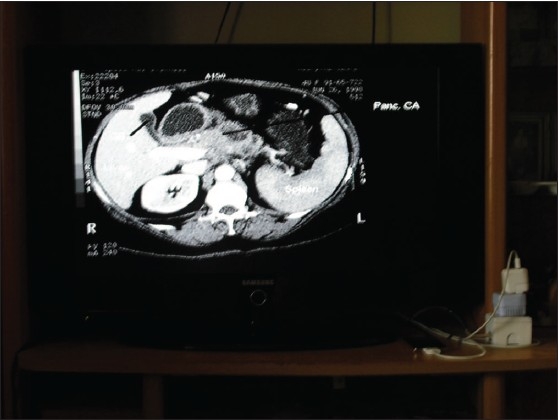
Photograph of the image viewer showing the iPOD connected to a TV display. The TV displays the test CT image (see text)
